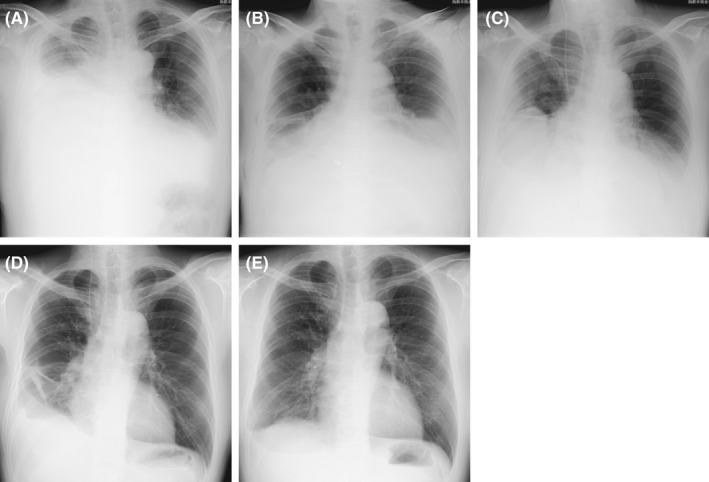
Chest radiography performed at the initial examination (A) showed a right side‐predominant bilateral opacity. Right‐sided pleural effusion was decreased following chest tube drainage on the right side (B). Right‐sided pleural effusion increased and left‐sided pleural effusion remained on day 7 (C), and a second bilateral thoracentesis procedure showed persistence of the *Enterobacter* infection. A chest radiograph obtained on day 21 (D), at which time meropenem was being administered, showed right‐sided scar‐like opacity. The opacity had nearly disappeared after 9 mo (E)
